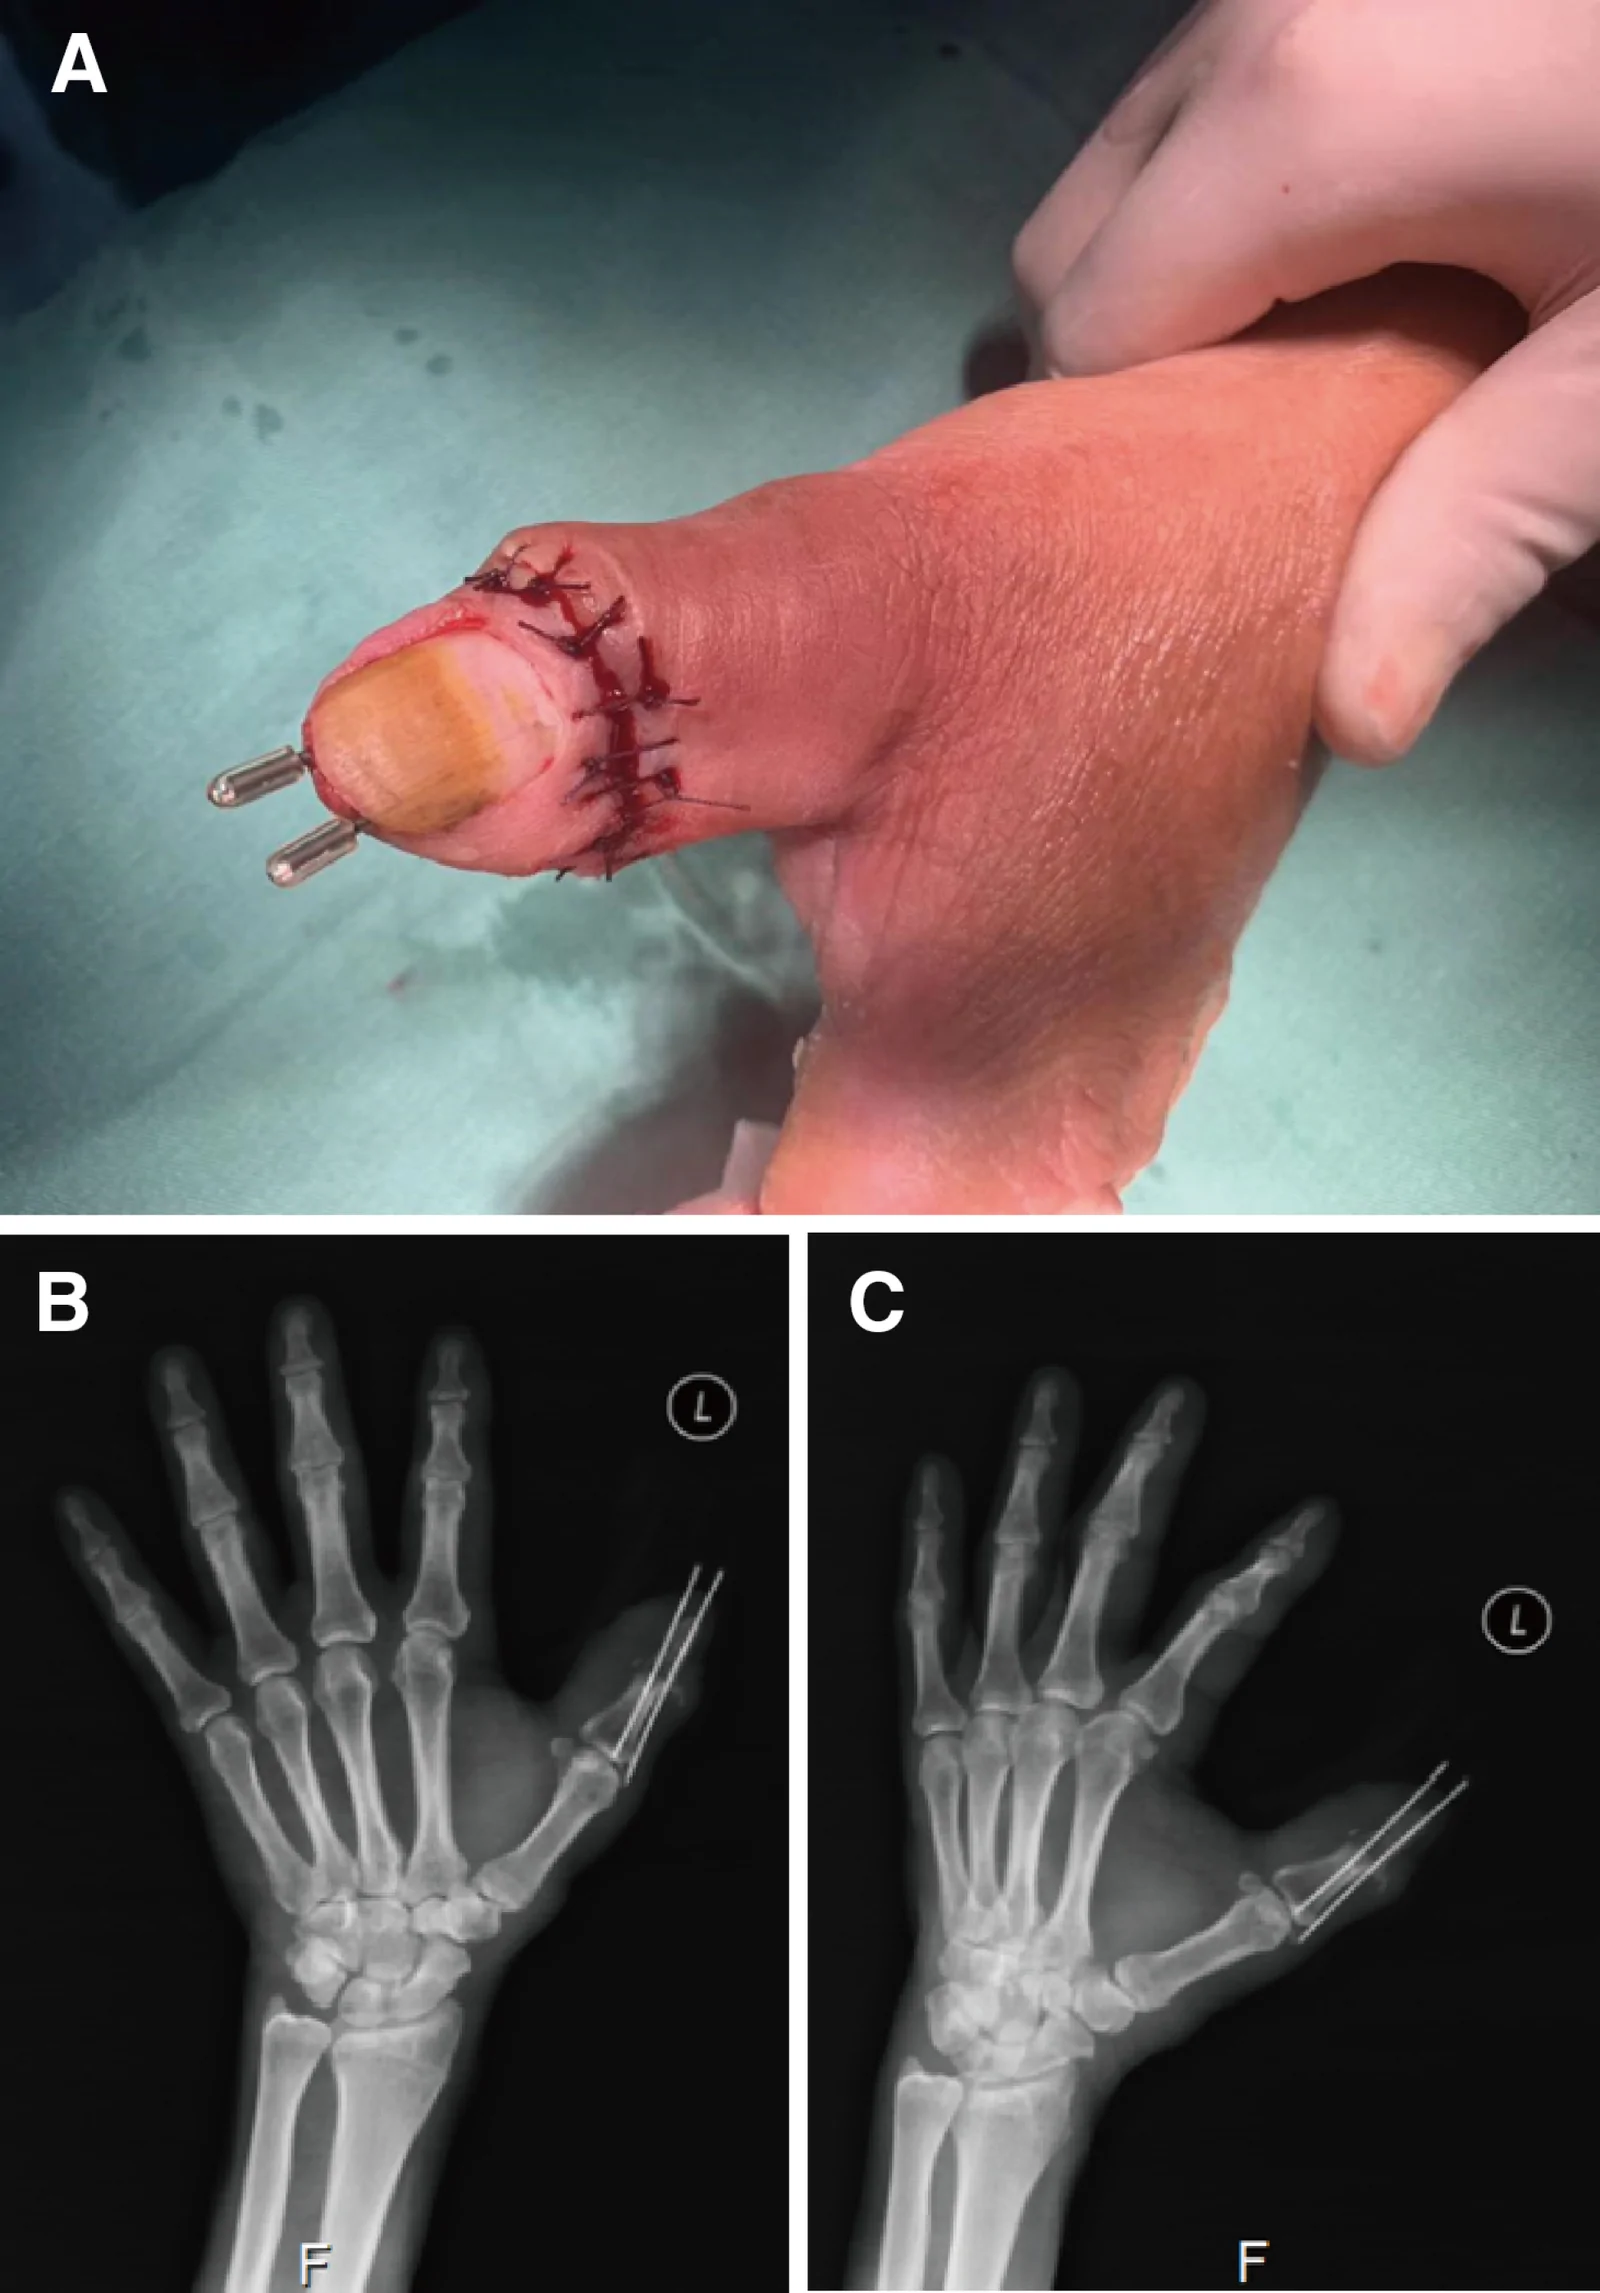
Postoperative photos (A) of bone cement removal and interphalangeal joint fusion with Kirschner wire internal fixation. Postoperative anteroposterior (B) and lateral (C) X-rays of the left hand.
